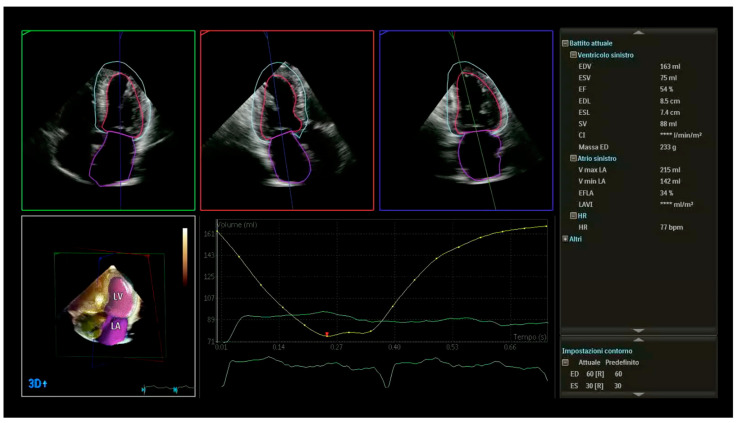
Example of 3D measurements (LV volumes and LV mass) obtained from automated DHM in a patient with cardiac amyloidosis and normal EF (54%). This advanced echocardiography allows for accurate, automated measurements of chamber volumes and function providing larger, more accurate LV volumes with good agreement with cardiac magnetic resonance when analyzed using the default settings of the boundary detection sliders (end-diastolic default position = 60/60; end-systolic default position = 30/30). LV myocardial mass (233 g) divided by the mean density of myocardium of 1.05 g/mL presents LV myocardial volume (221 mL). The ratio of SV (88 mL) to myocardial volume calculated the Myocardial Contraction Fraction (39%), significantly lower than the EF. LV: left ventricular; DHM: Dynamic Heart Model, Philips Healthcare, Andover, MA, USA; SV: stroke volume; EF: ejection fraction. DHM automatically measures chamber volumes throughout the cardiac cycle, resulting in LV time curve (yellow).
